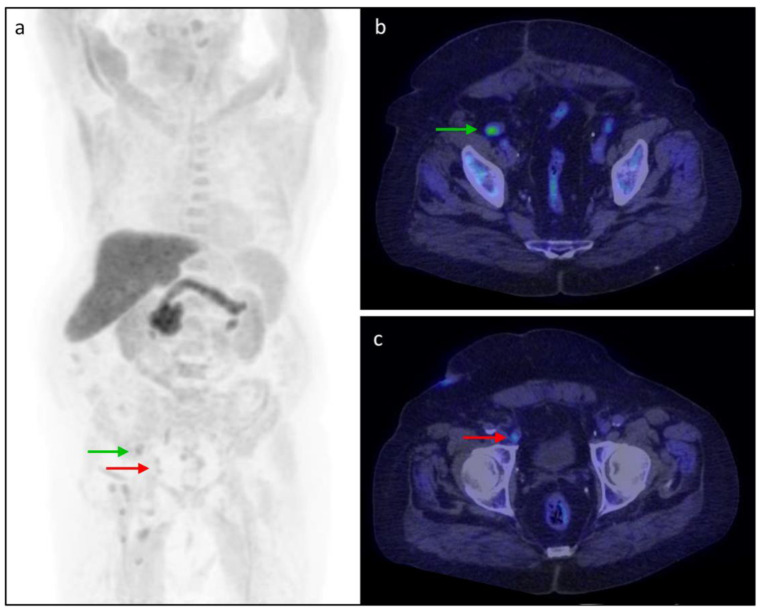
An 81-year-old patient with BCR after prostatectomy and Gleason Score 6 (3 + 3) underwent the [^18^F]fluciclovine PET/CT. PSA increased to 2.15 ng/mL with PSAdt of 13.6 months. The (a) MIP and (b,c) axial fused images showed recurrent lymph node lesions, namely (b) a right external iliac lymph node with SUVmax 5.6 (green arrow) and (c) a right deep inguinal lymph node with SUVmax 5.2 (red arrow).
